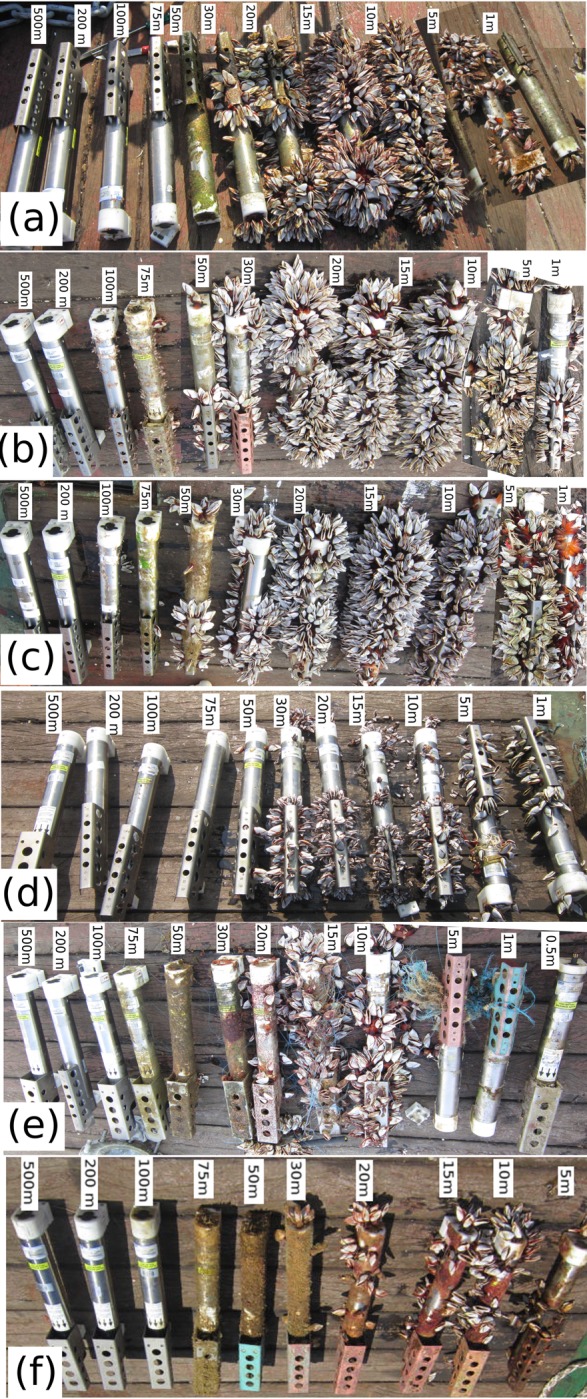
Conductivity-Temperature sensors retrieved from OMNI buoys (a) NEAS-AD06, (b) ECAS-AD07, (c) ECAS-AD08-A, (d) ECAS-AD08-B, (e) SEAS-AD10 and (f) SEAS-AD09. The labels in the figure indicate depth at which the CT sensors were deployed.
